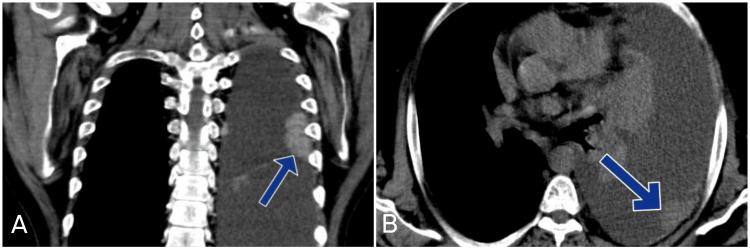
CT scan of the thorax showing soft tissue lesions of the pleura (blue arrows), suggestive of malignancy. (A) Coronal section, (B) Axial section.
